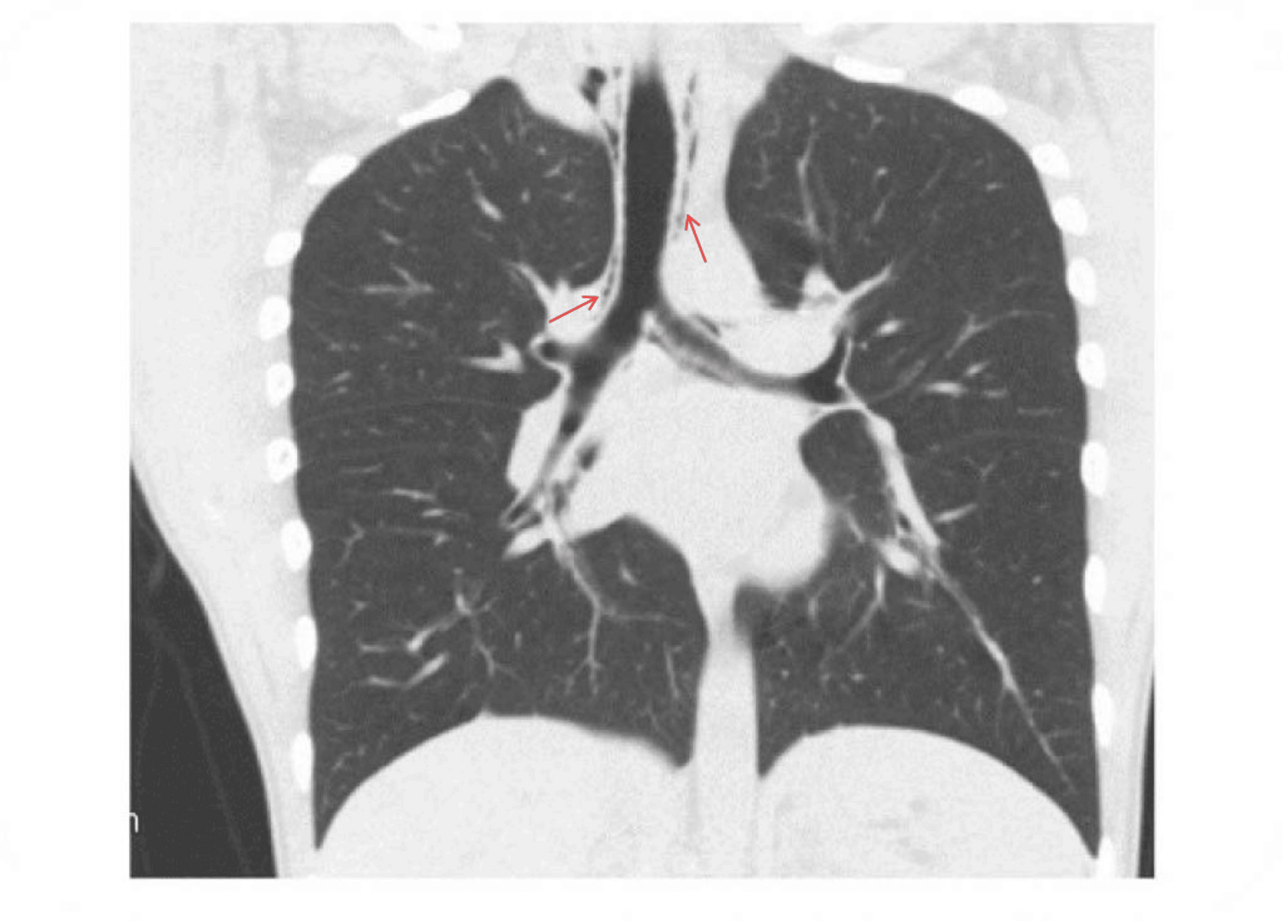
CT chest demonstrating free air in the mediastinal space indicative of pneumomediastinum CT: computed tomography
